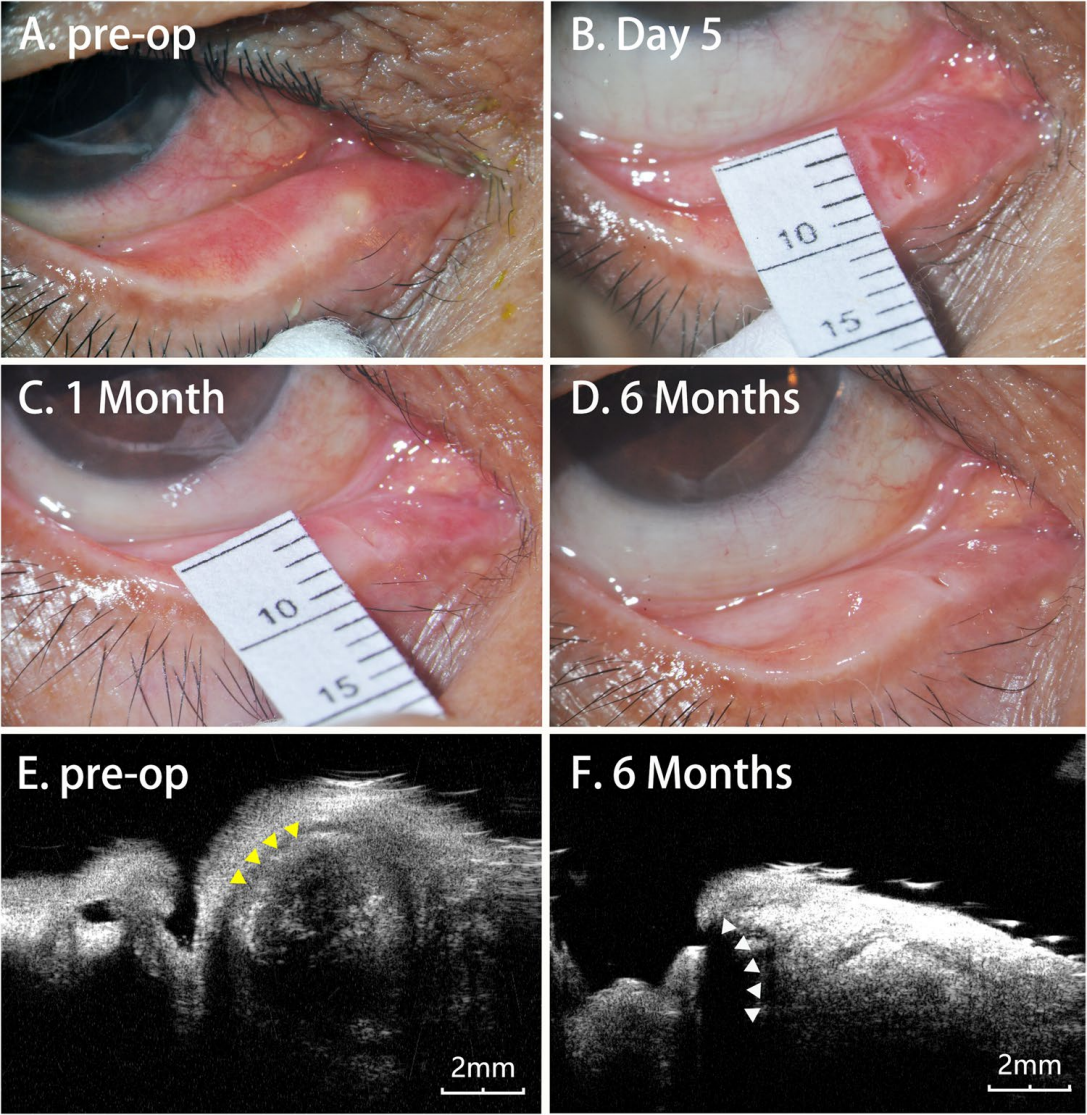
Preoperative and postoperative changes in the wound and UBM findings. A Mucopurulent discharge from the puncture was noted preoperatively. B Postoperative day 5, a 4-mm wound created by the CO2 laser was seen. C One month postoperatively, the wound was found to be healing smoothly with the adjacent epithelium to form a new punctum approximately 3 mm in length. D Six months postoperatively, the new punctum eventually stabilized in the form of a crack. E Preoperative UBM revealed the lower ectatic canaliculus near the punctum and thickened lumen wall (yellow arrowheads) accompanied by highly reflective intracanalicular concretions indicated by ultrasonic shadow. F Postoperative UBM showed the size of the canalicular lumen (white arrowheads) near the punctum at the follow-up visit 6 months after surgery
